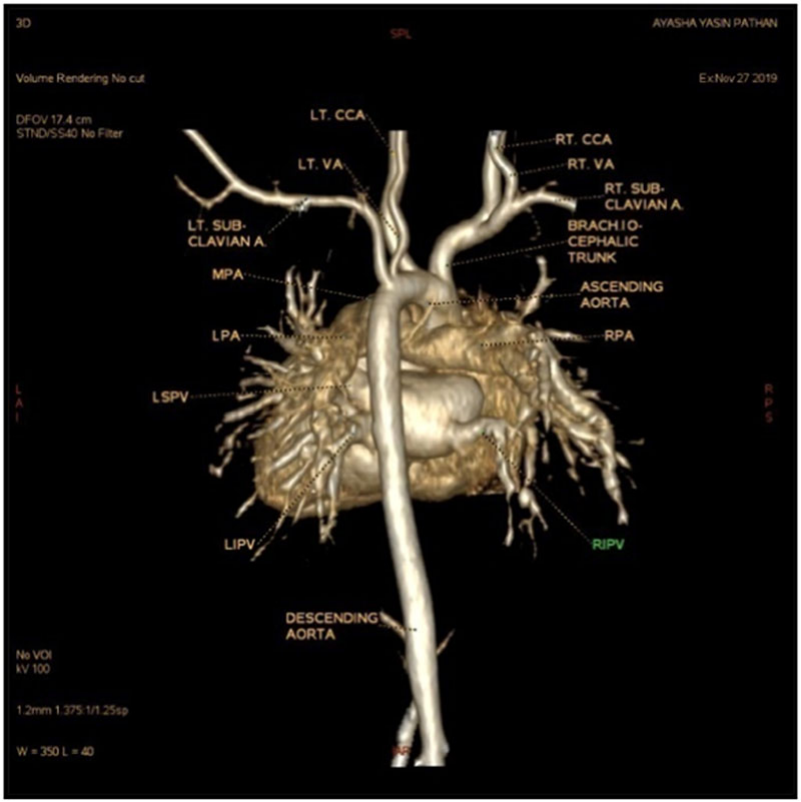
Volume rendering, anterior view, in dynamic cardiac computed tomography patient P4. CCA, common carotid artery; LIPV, left inferior pulmonary vein; LPA, left pulmonary artery; LSPV, left superior pulmonary vein; Lt., left; MPA, main pulmonary artery; RIPV, right inferior pulmonary vein; Rt., right; RVA, right vertebral artery (Image credits: Dr. Deepakkumar V. Mehta).
